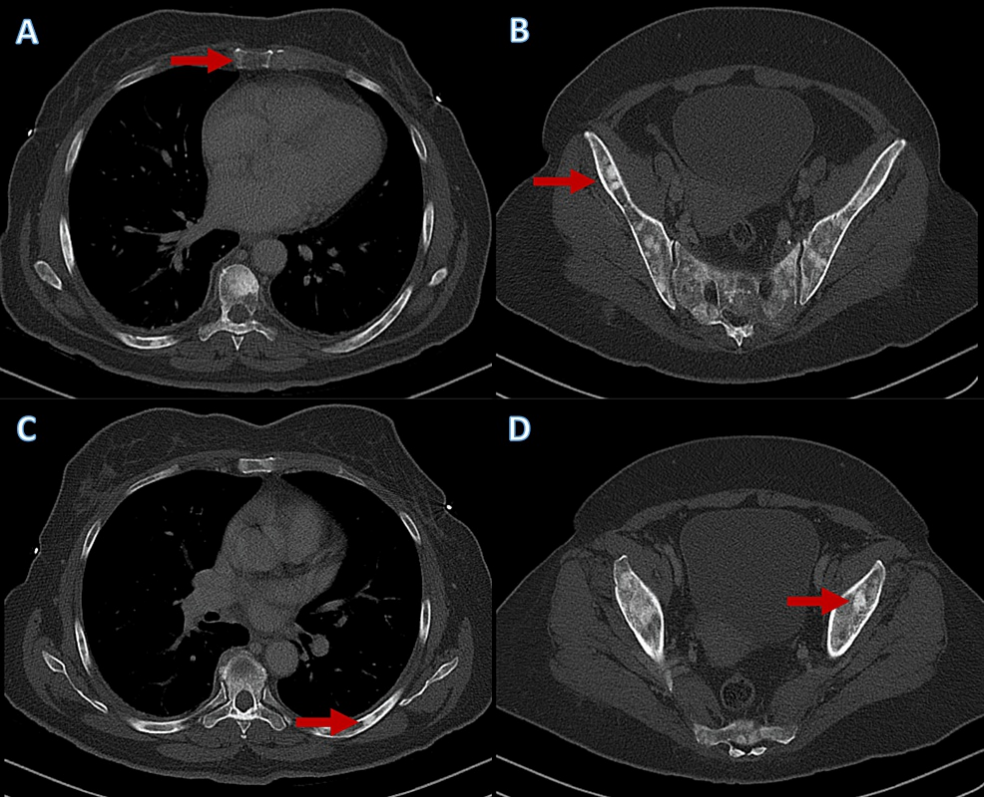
Multiple skeletal metastases Chest, abdominal, and pelvic computed tomography revealed multiple blastic and lytic lesions at the sternum (A), hip (B), ribs (C), and proximal femurs (D), compatible with diffuse bone metastases (examples highlighted by the red arrows)
